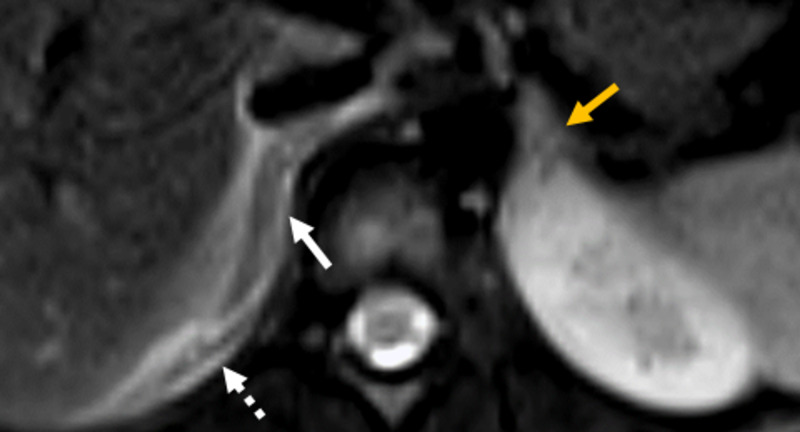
T2-weighted fat-suppressed images. Large, edematous, intermediate signal of the right adrenal gland (white arrow) with periadrenal and perirenal high signal indicating edema (white dashed arrow). The signal intensity of the right adrenal gland is slightly lower than the left. Of note, the left adrenal gland appears normal, without edema (golden arrow).
